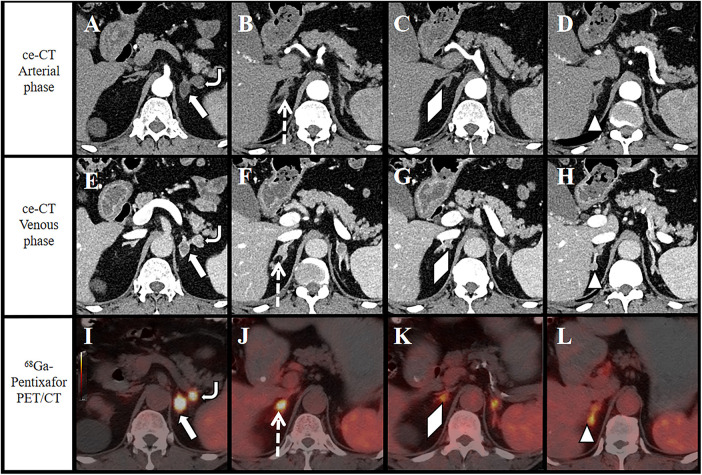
Three well-visualized adenomas are demonstrated on CT imaging. Contrast-enhanced(ce) CT arterial phase (A) and venous phase (E) revealed a quasi-spherical nodule in the left adrenal gland [17 mm; Hounsfield unit (HU) = 8.23; arrow] and a nodule in its lateral limb (19 mm × 14 mm; HU = 3.67; rounded arrow); ce CT arterial phase (B), and venous phase (F) showed an irregular nodule in the right adrenal body (16 mm × 11 mm; HU = 19.96, dashed arrow); all the 3 nodules exhibiting moderate enhancement with a rapid “fast-in-fast-out” pattern during arterial and venous phases. Fusion images from ^68^Ga-pentixafor PET/CT demonstrated significant radiotracer uptake in the bilateral adrenal. Three adenomas identified on CT demonstrated avid uptake on ^68^Ga-pentixafor PET/CT: (I), (arrow; SUVmax 31.30;rounded arrow; SUVmax 15.57); (J), (dashed arrow; SUVmax 7.50). Two metabolically active lesions were newly detected in the right adrenal gland and its medial branch: K, (triangular arrow; SUVmax 5.43; 10 × 9 mm; HU 19.21); (L), (diamond-shaped arrow; SUVmax 5.38; 10 × 7 mm; HU -1.69).
